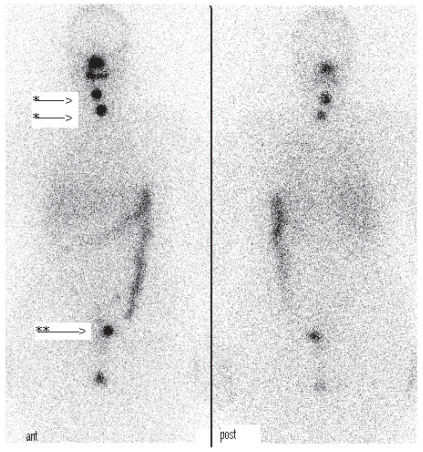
Total body scintigraphy eight days after 5550 MBq. ^131^I treatment. Two small spots in the region of the former thyroid are visible, probably remnant (*). In the pelvic region, there is pathological uptake visible just left of the bladder (). This could be residual tumor activity.
